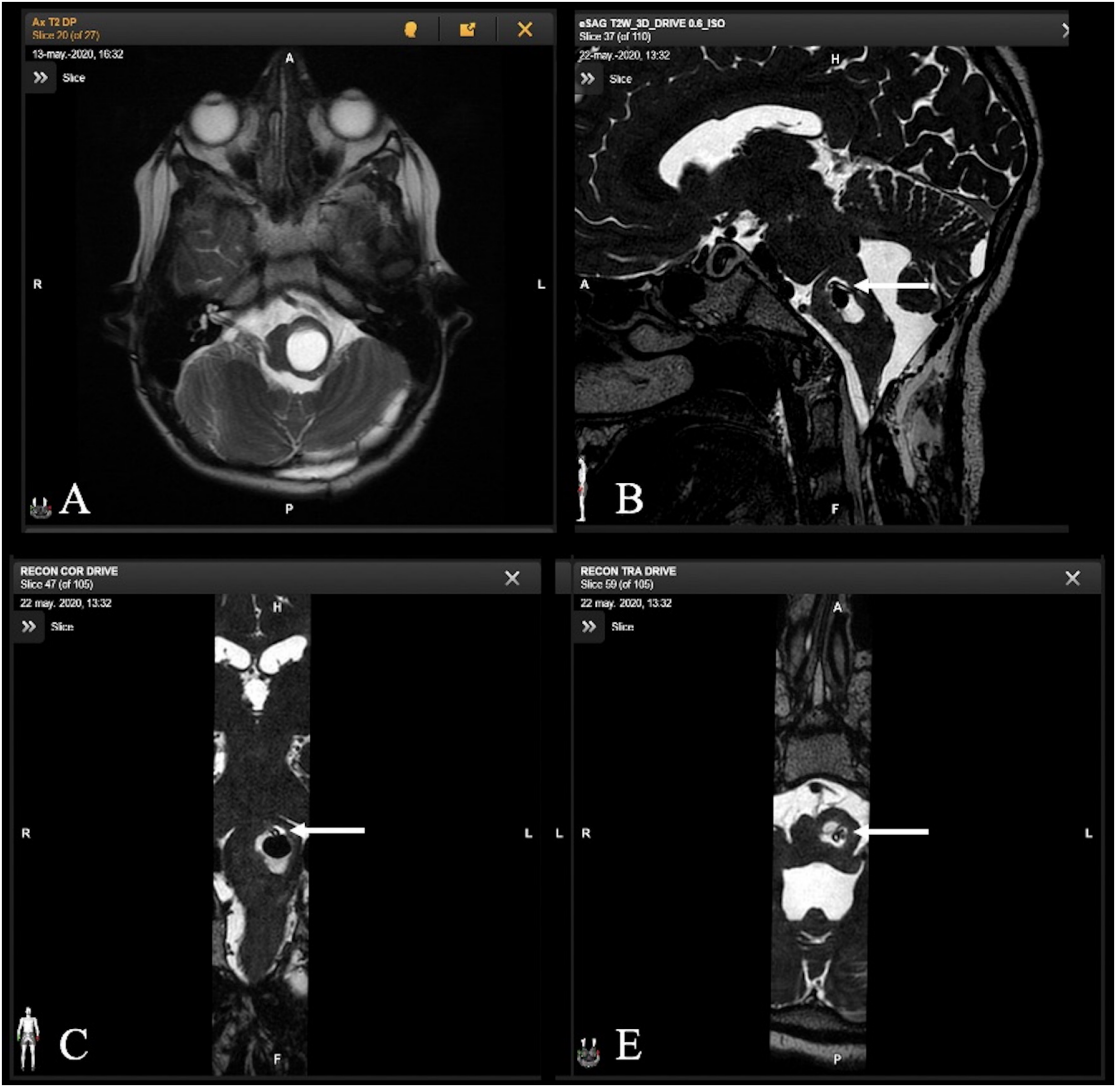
Axial T2-weighted MR image of a 13-year-old child with a focal brainstem tumor and a large cyst (A). Acquisition of intraoperative MR images as final controls with sagittal, axial, and coronal T2-weighted images shows correct placement of the catheter, indicated by the white arrow, within the tumor cyst component (B–D).
